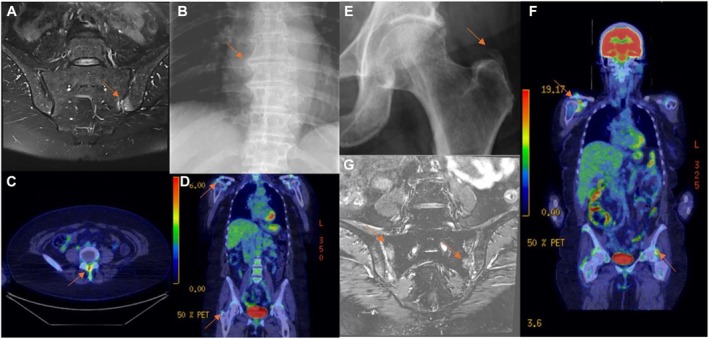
Images of two patients with polymyalgia rheumatica and spondyloarthritic involvement. (A–D) A 54‐year‐old female patient presenting with polymyalgia symptoms. The patient was HLA–B27 negative and had neither psoriasis nor familial history of SpA. Two years following glucocorticoid therapy, all treatments were discontinued without the initiation of any conventional synthetic disease‐modifying antirheumatic drugs. One year after cessation of treatment, the patient presented with recurrent inflammatory back pain, peripheral arthralgias, and elevated C‐reactive protein levels. Upon re‐evaluation, a diagnosis of SpA was confirmed. Subsequently, adalimumab therapy was initiated as part of the management strategy. (A) Bone marrow edema in sacroiliac joints on axial fat‐suppressed T2‐weighted magnetic resonance imaging (red arrow). (B) Unilateral osteophytes in thoracic vertebra (red arrow). (C) Interspinous FDG uptake on axial fused PET/CT (red arrow). (D) Shoulder and hip girdle FDG activity (red arrows) on coronal fused PET/CT, which is the hallmark finding of polymyalgia rheumatica. (E–G) An HLA–B27‐negative 66‐year‐old female patient presenting with polymyalgia symptoms with no SpA history. After glucocorticoid tapering, she had a relapse, and her therapy was changed to golimumab. (E) Trochanteric enthesitis on plain radiography (red arrow). (F) Shoulder and hip girdle involvement, a hallmark finding of polymyalgia rheumatica on coronal fused FDG‐PET/CT (red arrows). (G) Bilateral sacroiliac bone marrow edema on fat‐suppressed T2‐weighted magnetic resonance imaging (red arrows). FDG, fluorodeoxyglucose; PET/CT, positron emission tomography/computed tomography; SpA, spondyloarthritis.
